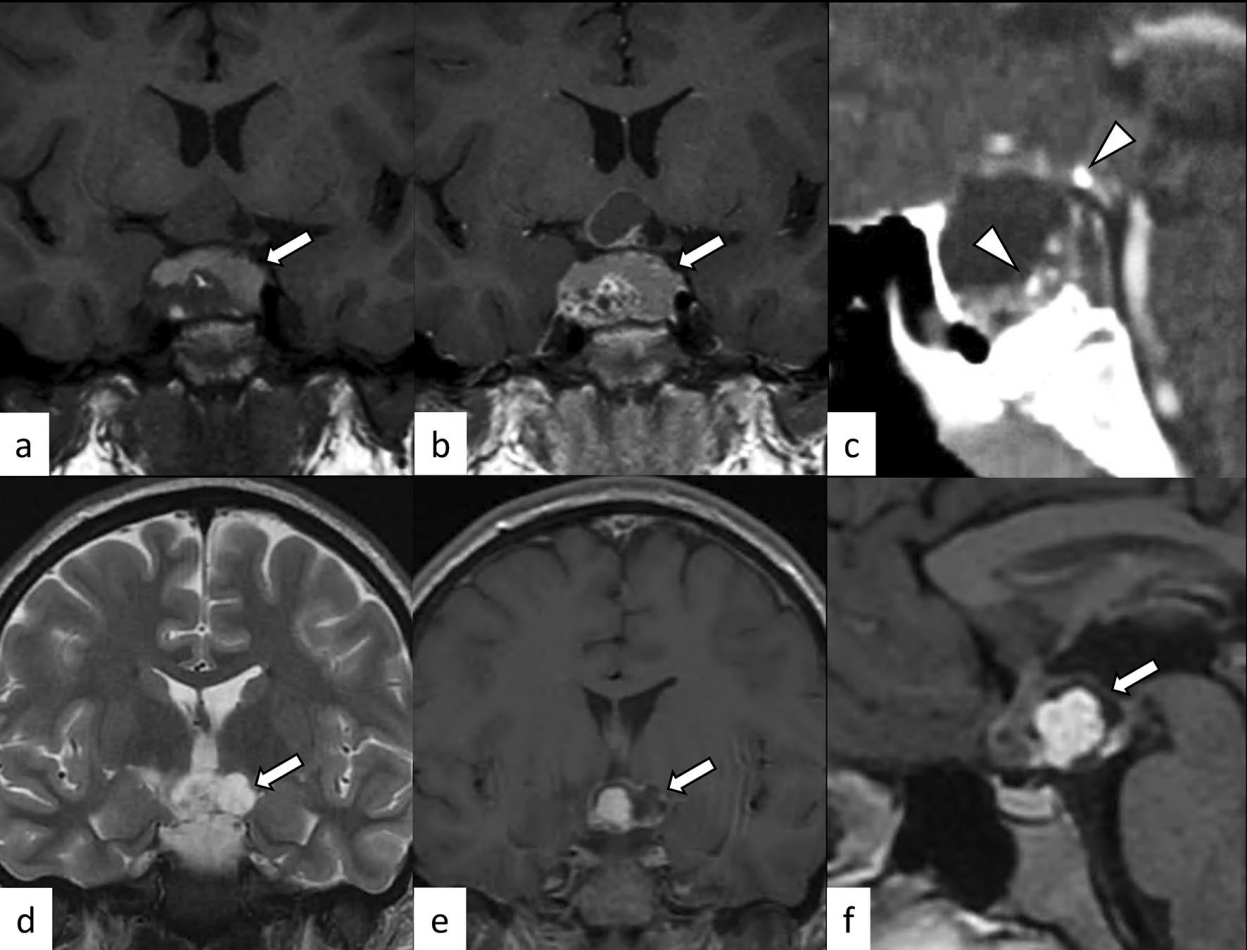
a–c, arrows A 14-year-old boy with adamantinomatous craniopharyngioma. MRI shows a mass with heterogeneous cystic signal intensity with contrast-enhanced components (a, T1-weighted coronal image; b post-contrast T1-weighted coronal image). Calcifications are observed on contrast-enhanced CT (c arrowheads). (d–f, arrows) A 21-year-old man with papillary craniopharyngioma. There is a suprasellar mass with heterogeneous high-to isointense solid components on T2-weighted coronal image d with strong contrast enhancement e, f and peripheral multicystic components
